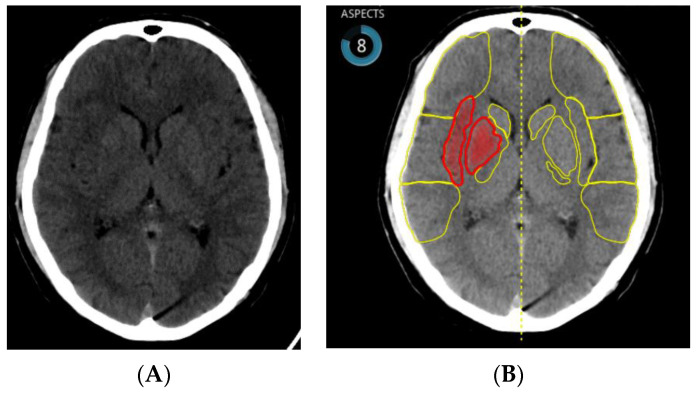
(A) NCCT brain scan—ischemic changes in territory of the right middle cerebral artery (insula and basal ganglia) and (B) identical patient—ischemia detected by artificial intelligence (ASPECT score = 8).
